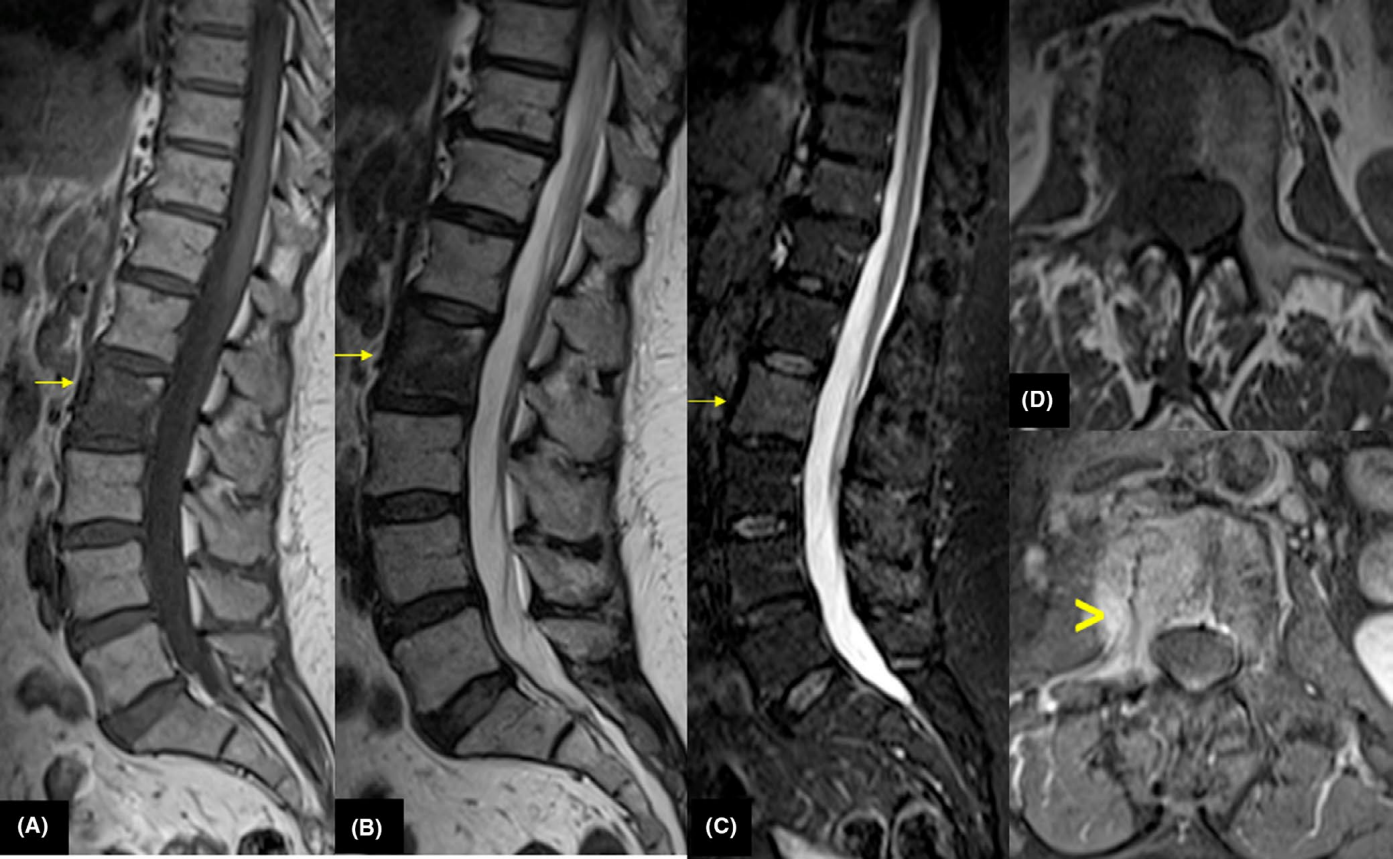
MRI sagittal T1 WI (A), sagittal T2 WI (B), sagittal STIR WI (C), axial T1 WI (D), and axial T1 fat sat gadolinium show decreased signal on T1 WI and high signal on T2 and after gadolinium administration throughout vertebral bodies (arrow) with soft‐tissue mass (arrowhead)
